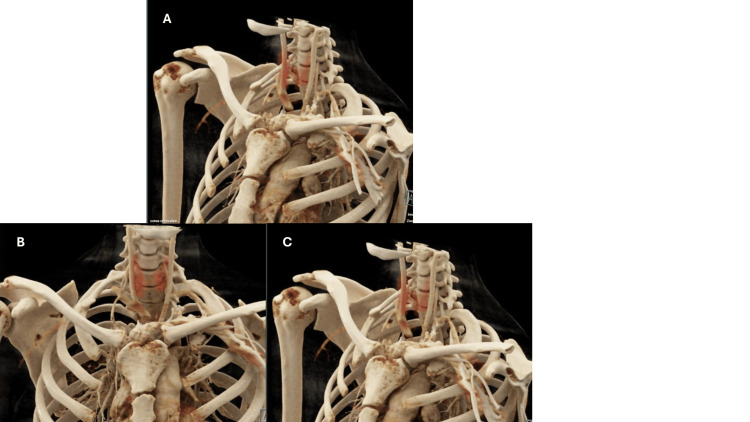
CT scan with three-dimensional reconstruction of the thoracic outlet before the cervical rib resection
